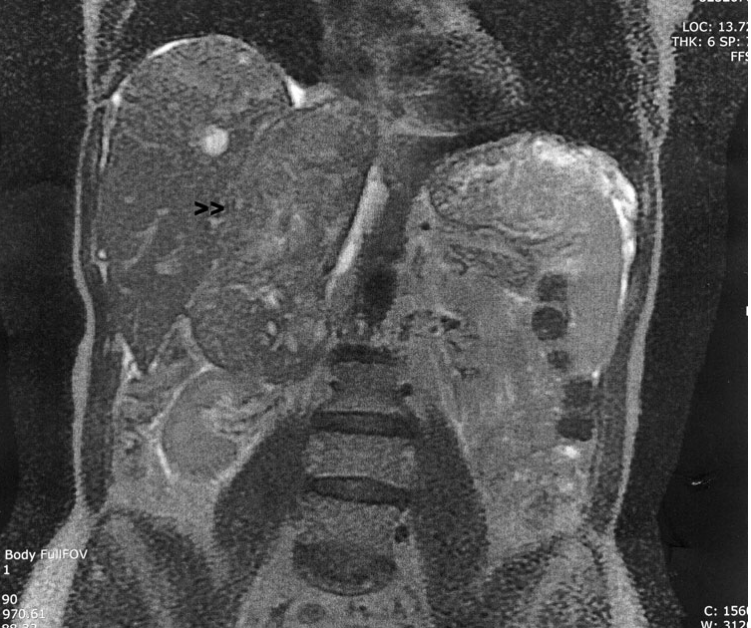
Sagittal view of abdominal MRI. Tumor (arrow) extends from the superior pole of the right kidney to the right atrium.
